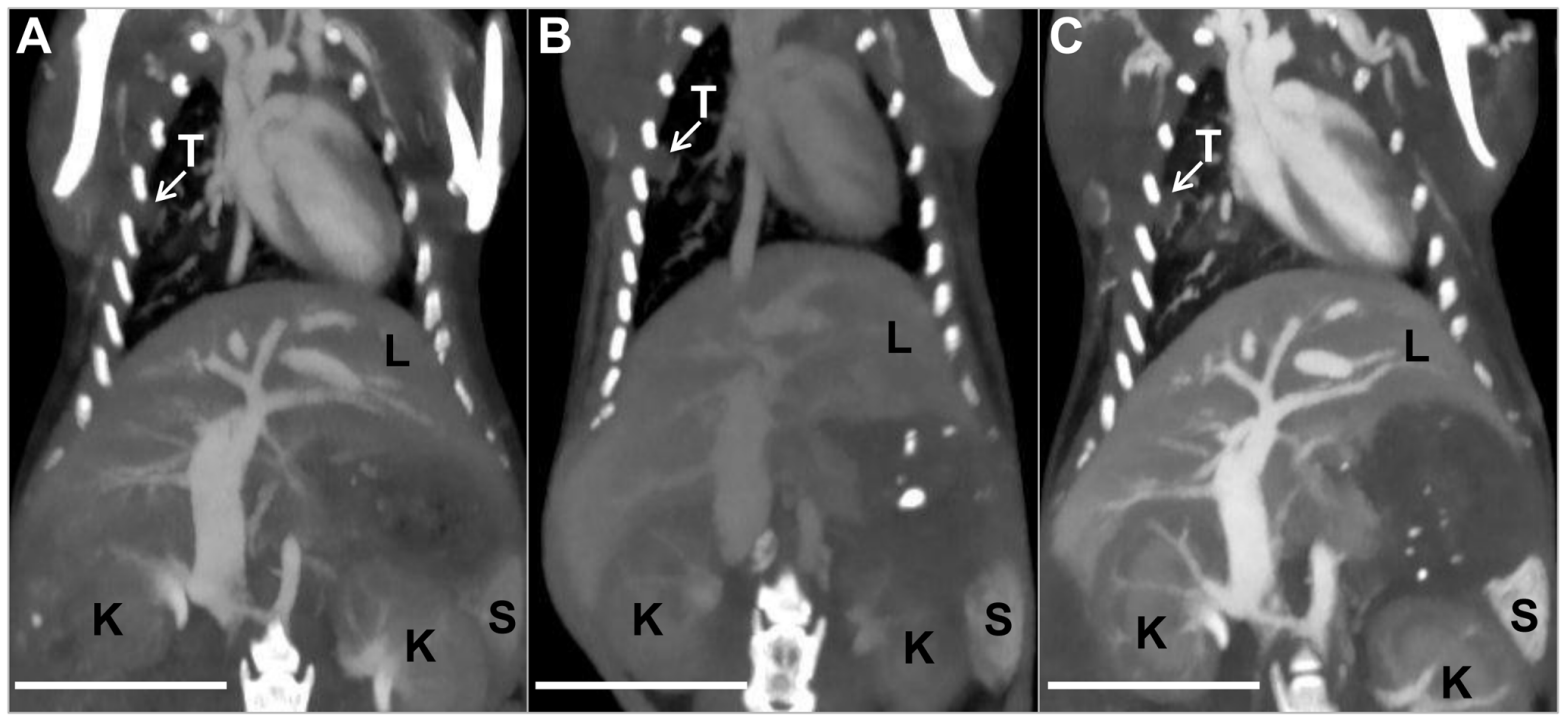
Thick slab maximum intensity projections of the 80 kVp CT datasets following bilateral filtration. CT scan 1 is shown in (A), CT scan 2 in (B) and CT scan 3 in (C). Tumor is marked “T,” liver is marked “L,” spleen is marked “S.” and kidneys are marked “K.” The images are all windowed between −300 and 1200 HU. The scale bar represents 1 cm in all panels.
